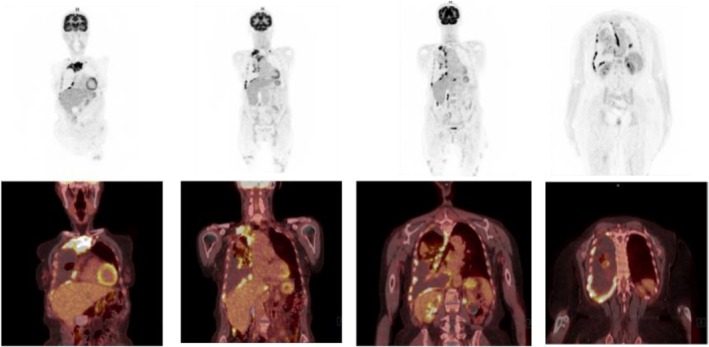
18F‐FDG PET/CT images showing multiple areas of pathological uptake. Maximum intensity projection images (top row) highlight widespread hypermetabolic lesions. Corresponding fused PET/CT images (bottom row) demonstrate intense FDG uptake in multiple regions, including thoracic and abdominal sites, indicating metabolically active disease.
